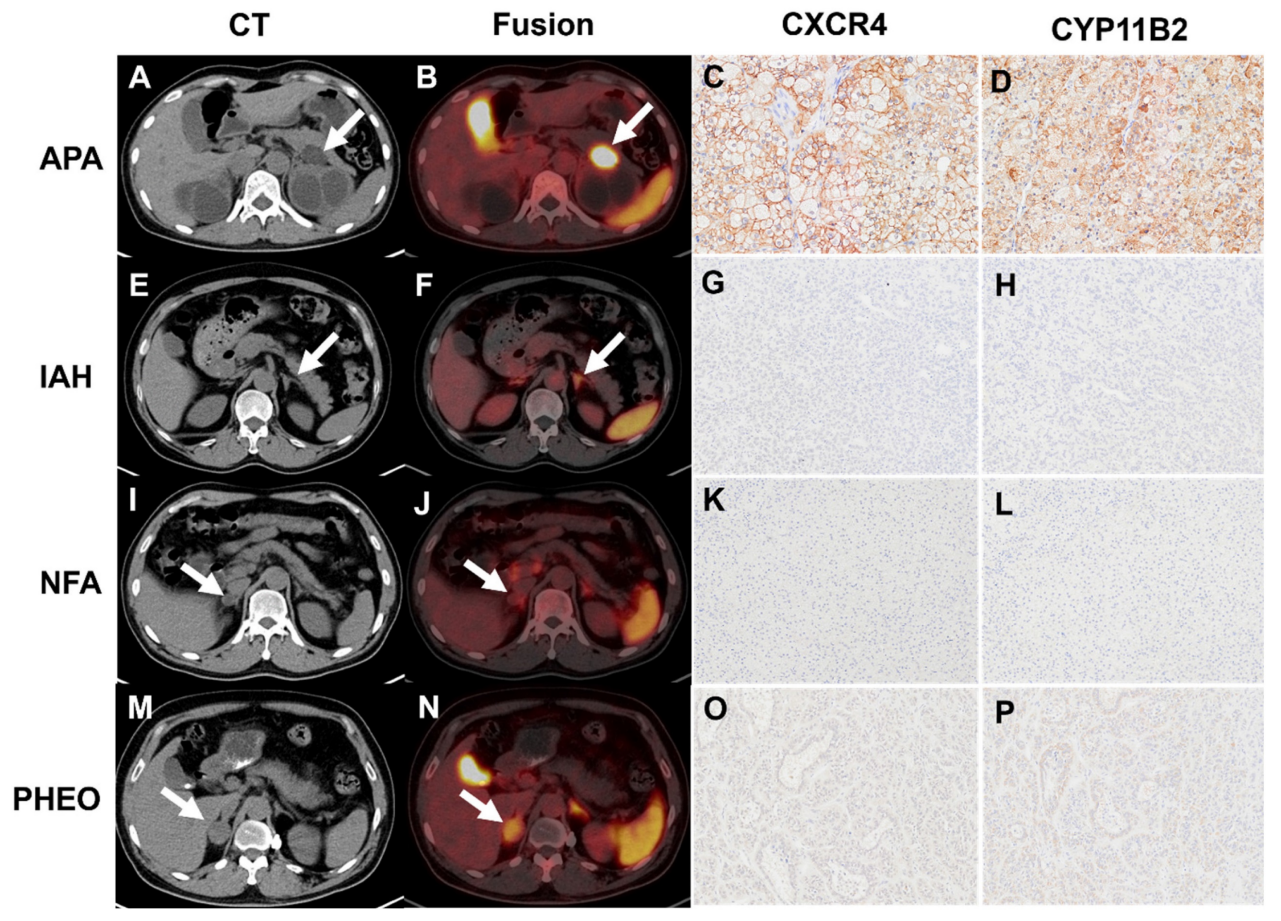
A-D: A true positive uptake (SUVmax 50.48, white arrow) was observed in one APA lesion with strong expression of CXCR4 and CYP11B2. E-H: [^18^F]AlF-NOTA-pentixather PET/CT was true negative in IHA (SUVmax 10.65, white arrow) with no elevated expression of CXCR4 and CYP11B2. I-L: Representative [^18^F]AlF-NOTA-pentixather PET/CT of true-negative NFA lesion (SUVmax 2.80, white arrow) with no elevated expression of CXCR4 and CYP11B2. M-P: [^18^F]AlF-NOTA-pentixather PET/CT of PHEO (SUVmax 11.30, white arrow) with a few expressions of CXCR4 and CYP11B2.
